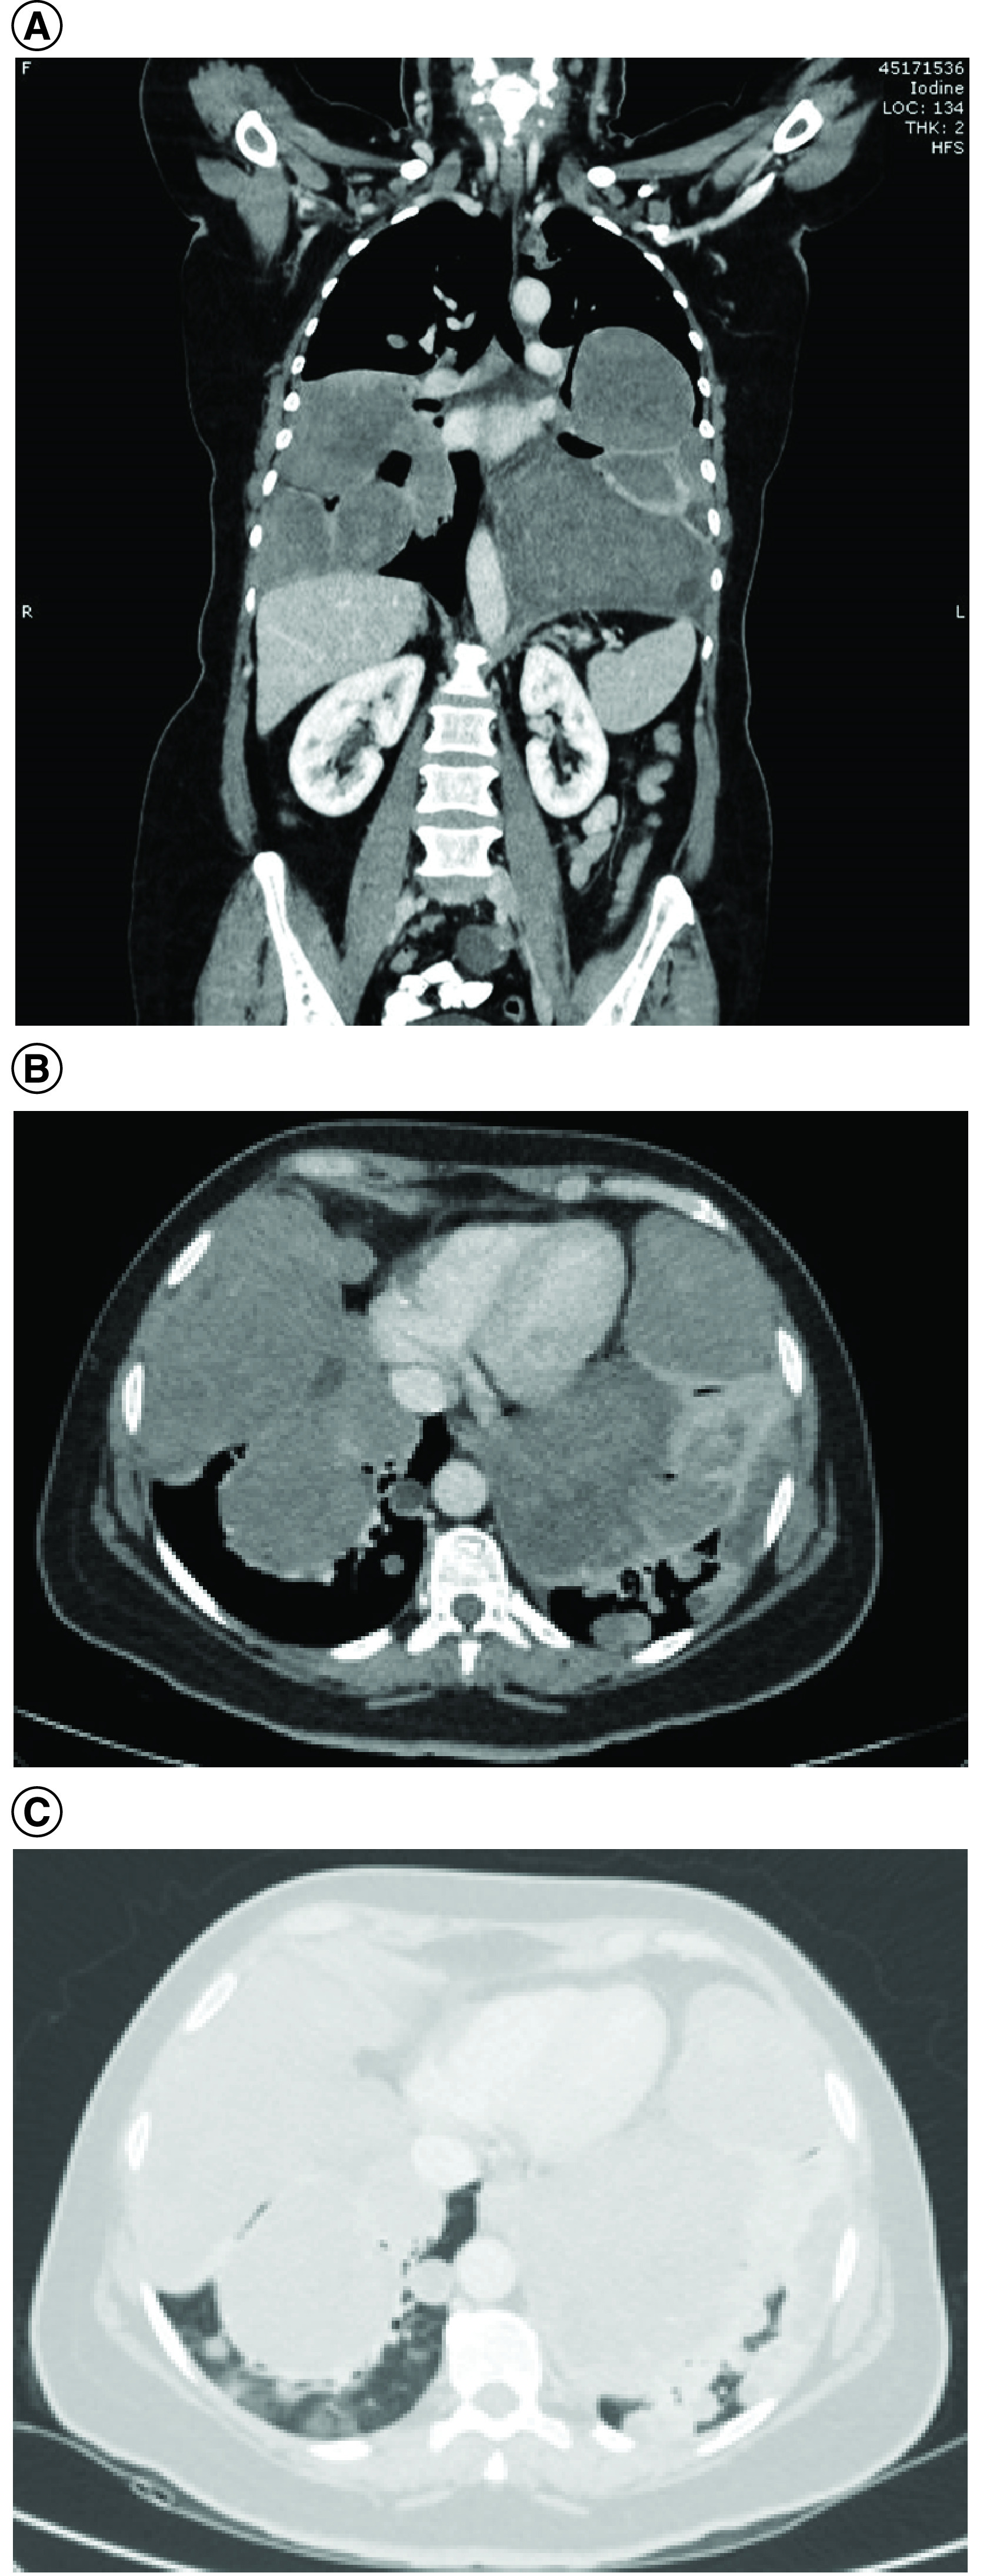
Computed tomography scan showing multiple enlarged bilateral pulmonary nodules. (A) Coronal soft tissue window. (B) Axial soft tissue window. (C) Axial lung window.
